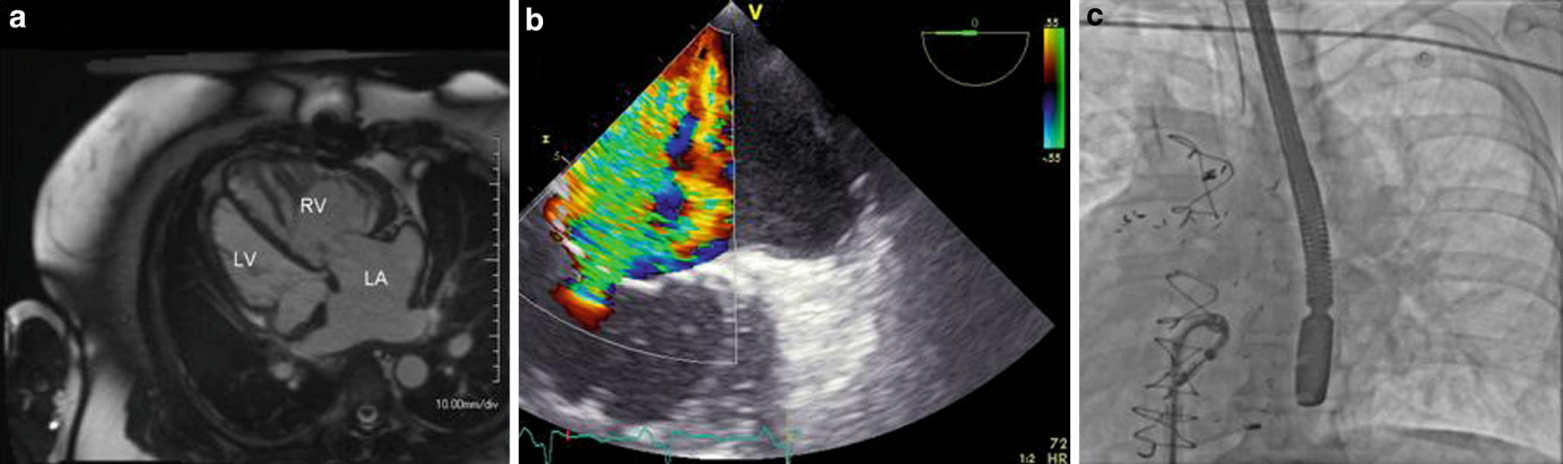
Magnetic resonance imaging showing a ccTGA diagnosis. The hypertrabeculated system ventricle is a morphological right ventricle (a). Echocardiographic images showing severe tricuspid regurgitation pre-MitraClip® (b). Fluoroscopy showing the delivery system and clip. Note the dextrocardia with apex to the right (c)
